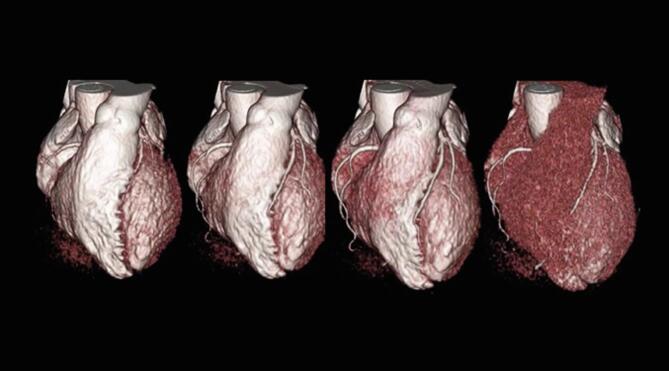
A volume rendering of dynamic coronary CT (computed tomography) angiography for a case of heterozygous familial hypercholesterolemia in a woman in her 40 s. This depiction shows the first pass of contrast media from the heart cavities into their normal coronary arteries. Herein, the flow is shown to be travelling from the left to the right over time.
